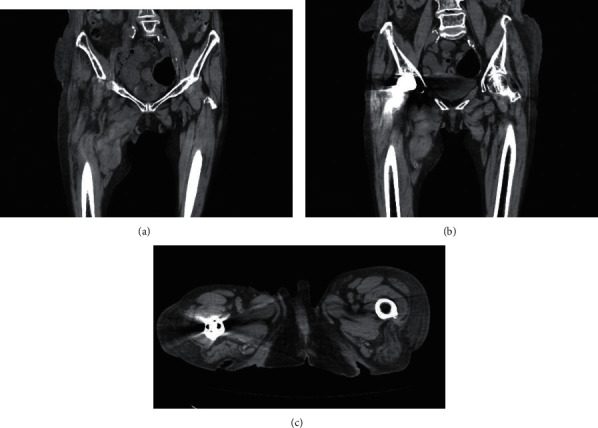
(a) CT on POD 4 showing hematoma spreading from the front of the right pubis to the inner aspect of the thigh. (b) CT on POD 9. Hematoma progression was observed without expansion. (c) CT on POD 53. The hematoma and subcutaneous bleeding disappeared.
